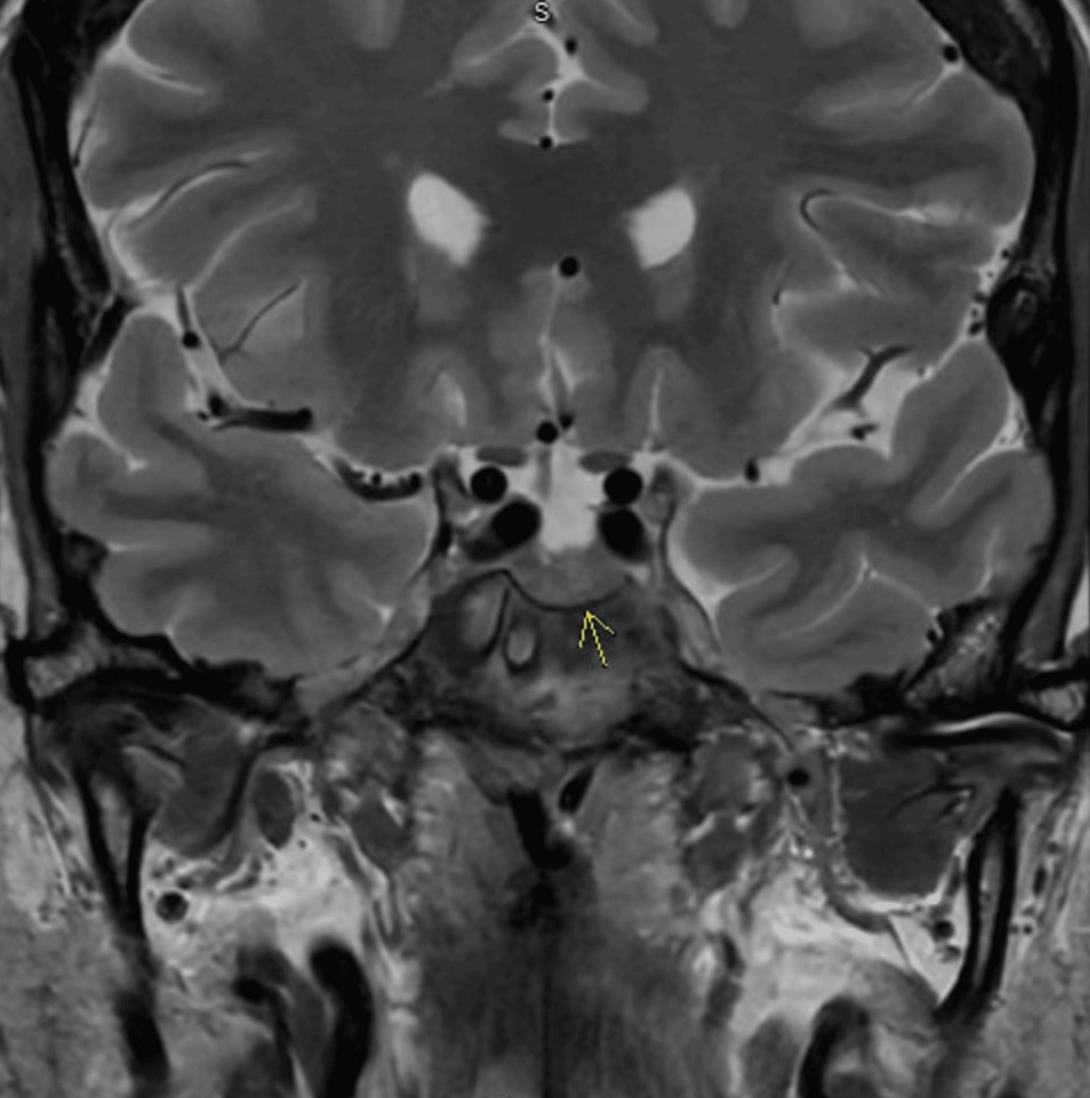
MRI sella with contrast. Arrow points to the area of hypophysitis
